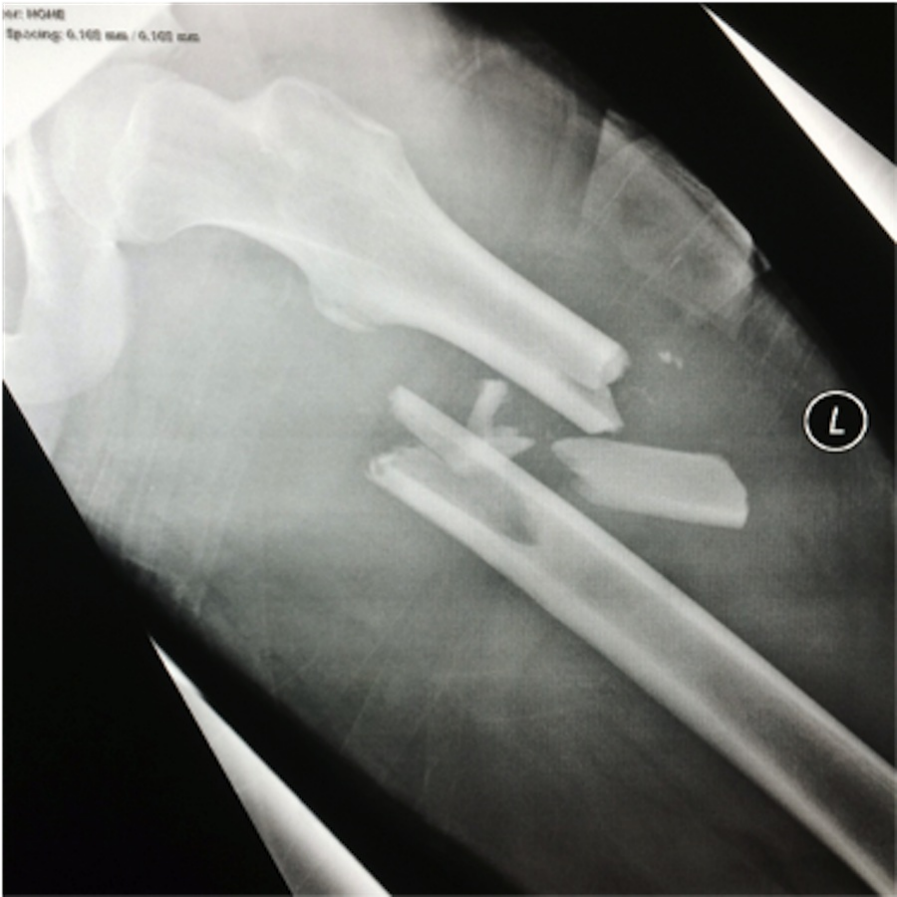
Radiograph of left femur demonstrating a comminuted mid-shaft fracture (Source: K Chan)
